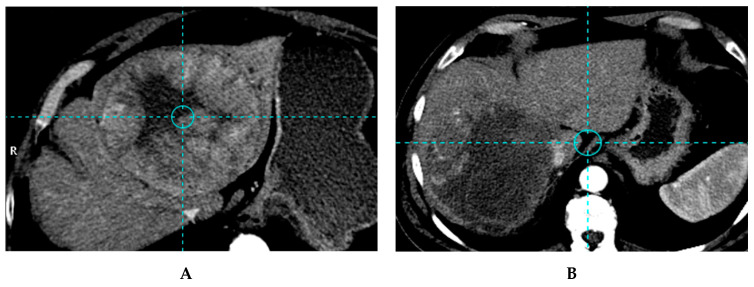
Example of a patient treated with a personalised dosimetry approach, with a lesion accurately treated (tumor dose higher than the 205 Gy threshold). (A) Based CT image of 15 cm unifocal right HCC with the invasion of the veina cava in a 64-year-old patient with good performance status (ECOG 0) and liver functions (Child Pugh A5). The patient received a treatment of the right lobe with 7.06 GBq of ^90^Y glass microspheres using the personalized dosimetry approach delivering 294 Gy to the large lesion and 173 Gy to the right lobe. CT image, 3 months after SIRT, partial response of the huge lesion was obtained using EASL criteria, as no recurrence on the left lobe and no extra hepatic spread (B). The patient was still in good performance status (ECOG 0) and liver functions (Child Pugh A5) and a right hepatectomy was performed with veina cave reconstruction. At last follow-up evaluation, 22 months after SIRT, the patient was still in complete response.
